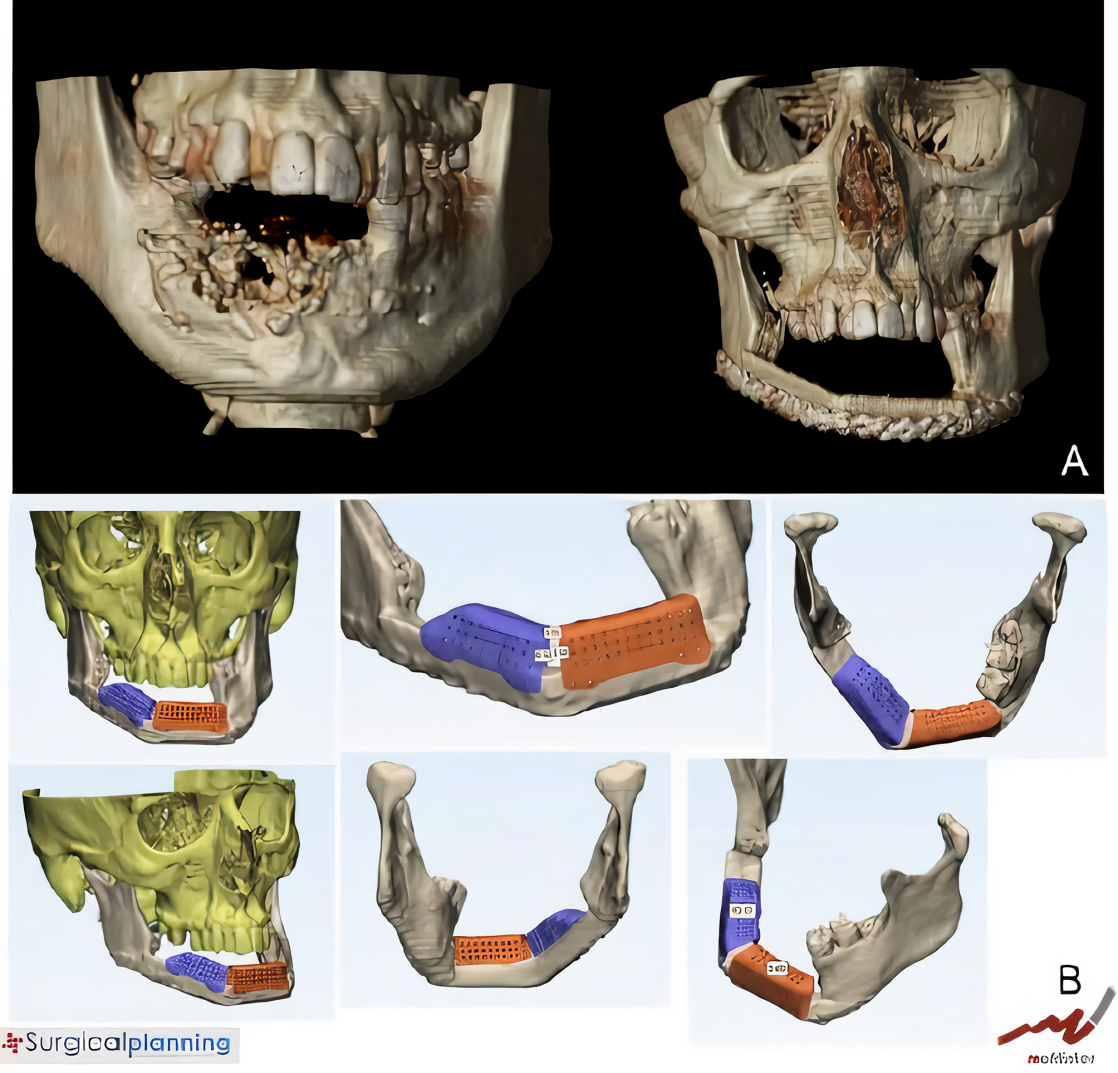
(A) CT Scan showing destruction of the mandibular symphysis, segmental mandibulectomy from left mandibular body to right mandibular angle and immediate reconstruction with fibula free flap. (B) VSP for vertical reconstruction of the fibula with two CAD/CAM titanium meshes adapted to the two segments of the fibula flap.
